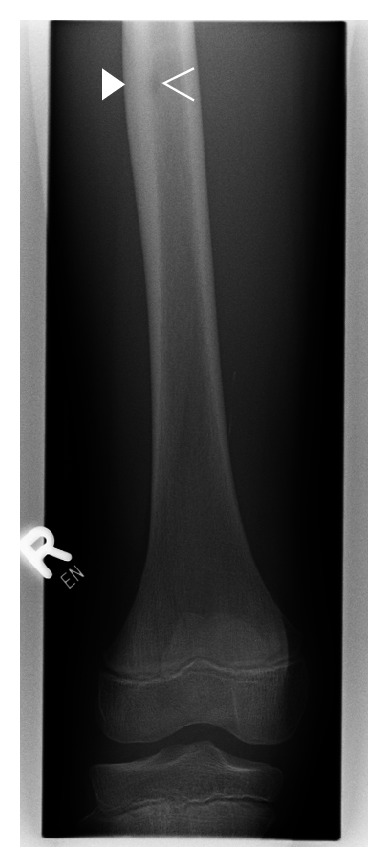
X-ray of the knee and the distal femur shows an osteoid osteoma-like lesion with typical cortical reaction (solid arrowhead) and a suspected central lucency (open arrowhead).
